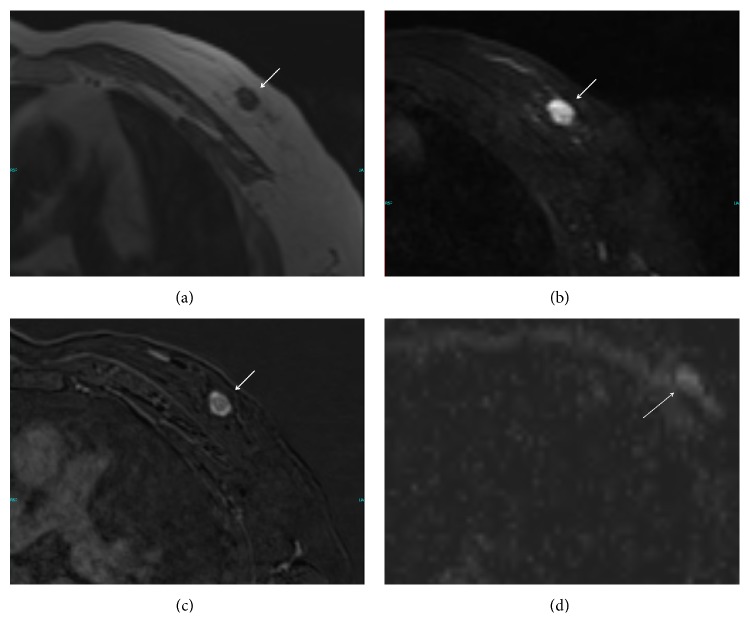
Magnetic resonance imaging findings of the breast mass. (a) T1-weighted spin echo MR image shows a focal mass with low signal intensity and smooth borders. (b) The fat-suppressed fast spin echo T2-weighted MR image shows a focal mass with high signal intensity. (c) Gd-enhanced fat-suppressed T1-weighted MR image shows strong heterogeneous enhancement of the mass and linear areas of low signal intensity in keeping with internal septations. (d) ADC value of the mass.
